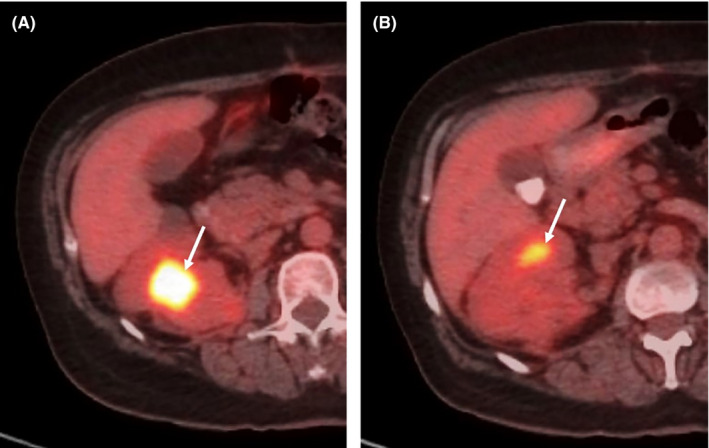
A and B, Follow‐up axial FDG PET/CT scan images of right renal mass and mediastinal lymph nodes in a patient with renal Rosai‐Dorfman disease. (A) The tumor in the right renal pelvicalyceal system (arrow) shows increased uptake (SUV_max_: 13.3) as well as (B) few renal cortical foci (arrow) with mild uptake (SUV_max_: 6.3)
